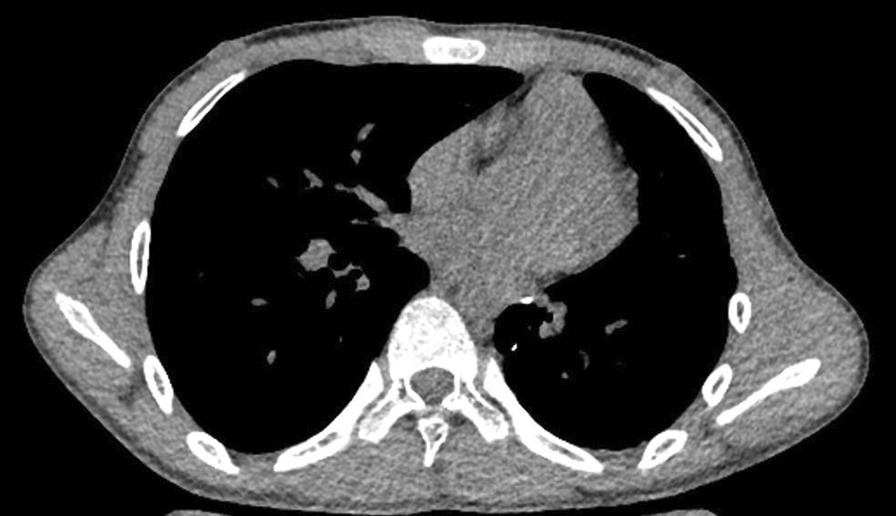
Postoperative review of computed tomography showed no recrudescence of PAVF. (Mediastinal window)
